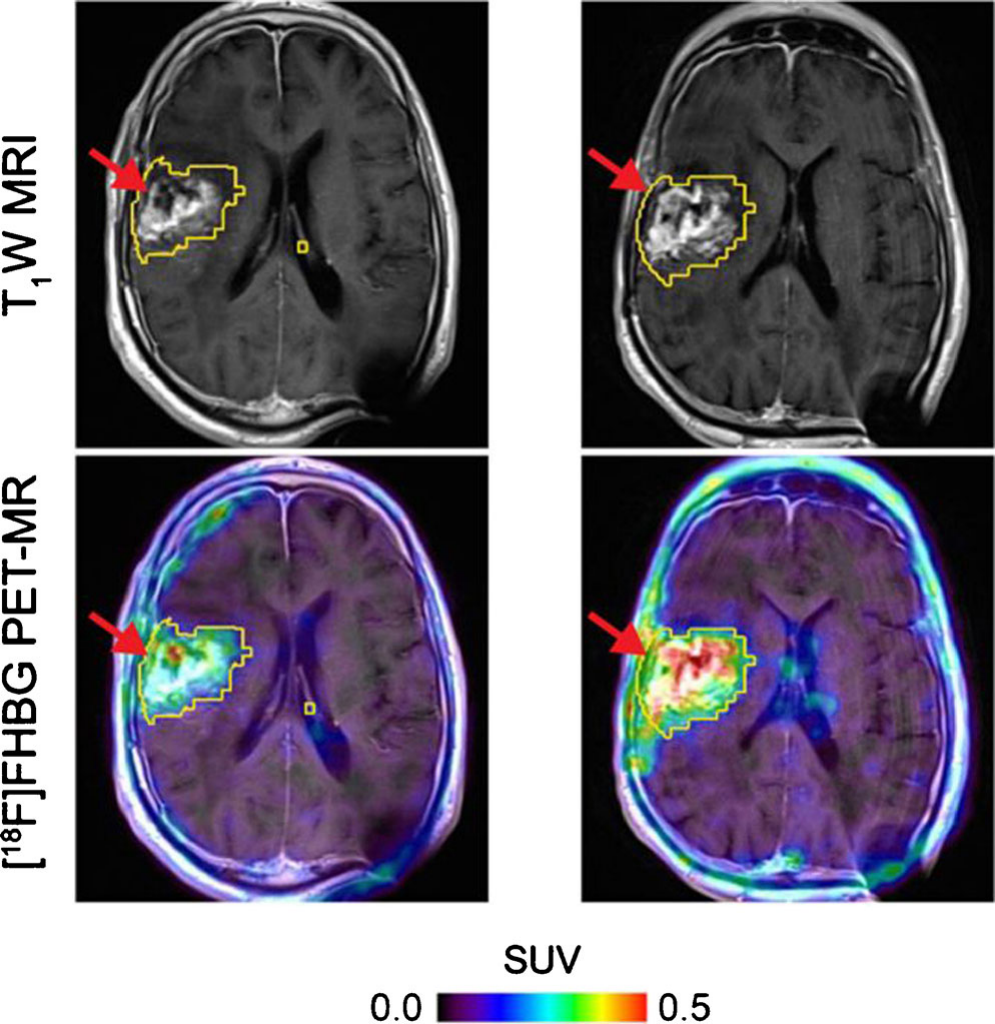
Tumor-directed CAR-T cell PET imaging with [^18^F]FHBG. [^18^F]FHBG PET imaging was performed in a patient with a recurrent right frontoparietal glioblastoma a before and b 1 week after tumor-specific CAR-T cell infusions. Allogeneic CAR-T cells and IL-2 were injected intratumorally (*red arrows*). Tumor recurrence was monitored by T_1_-weighted (T_1_W) MRI (top panels). [^18^F]FHBG PET images were fused with MR images (*bottom panels*), and three-dimensional (3D) volumes of interest were drawn using a 50 % [^18^F]FHBG SUV_max_threshold, outlined in *yellow* (adapted from [80]).
